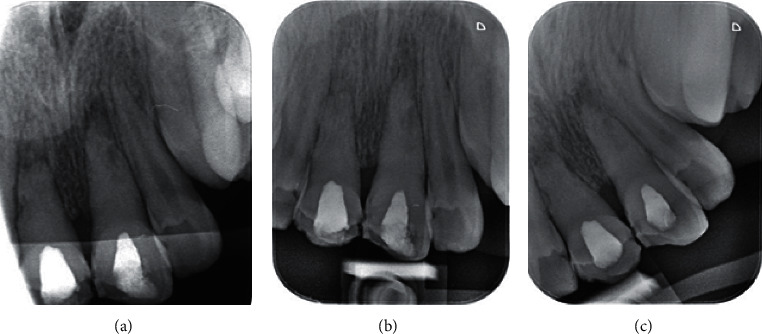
(a) Periapical radiograph taken at 1 month after the application of powdery calcium hydroxide; (b) periapical radiograph taken after 2 months; (c) periapical radiograph taken after 3 months, showing sound periodontal ligament.
